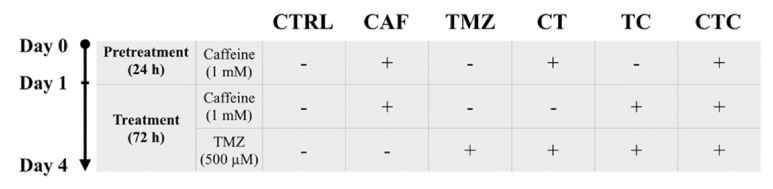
Demonstration of experimental strategies. CTRL: control; CAF: caffeine alone; TMZ: temozolomide alone; CT: temezolomide with caffeine pretreatment; TC: combined treatment of temozolomide and caffeine; and CTC: combined therapy with caffeine pretreatment.
